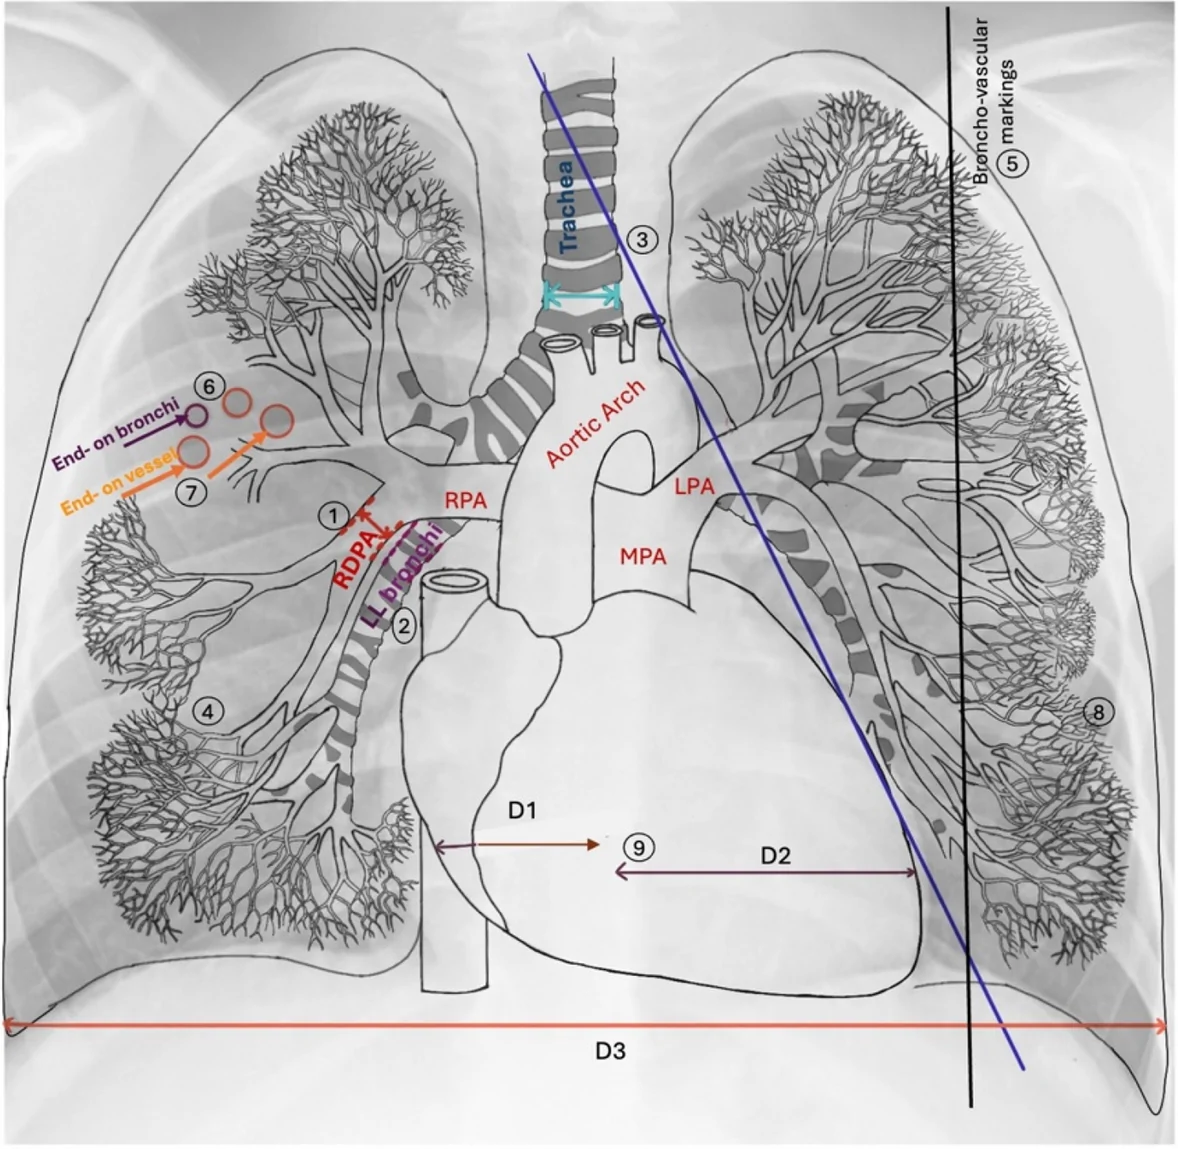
Pictorial representation of the defining features of pulmonary vascularity on chest radiograph. (1) Right descending pulmonary artery (RDPA) and tracheal diameter. (2) RDPA and right lower lobe bronchi. (3) Tangential line from aortic knuckle to left heart border. (4) Individual peripheral vessels. (5) Bronchovascular marking. (6) Number of end-on vessels. (7) Size of individual end-on vessel and respective end-on bronchi. (8) Opacity of lung fields. (9) Cardiothoracic ratio (D1 + D2/D3). LPA, left pulmonary artery; MPA, main pulmonary artery; RPA, right pulmonary artery
